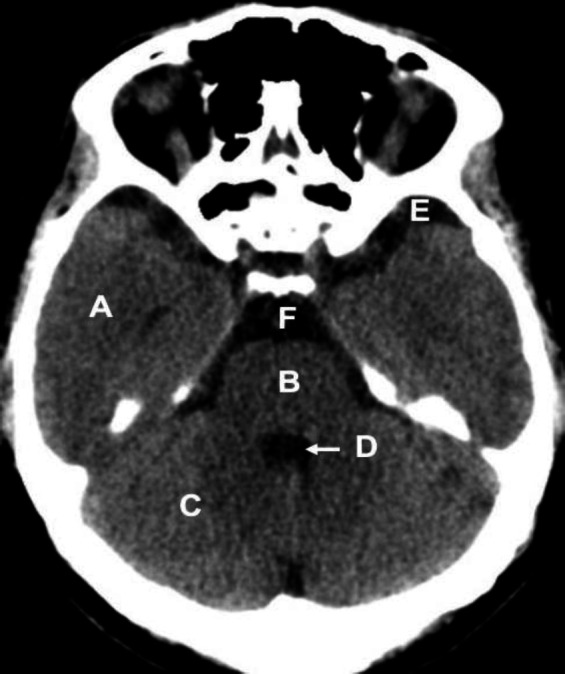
Identify the normal anatomical structures labelled A-F on a non-contrast CT brain of a middle-aged man
